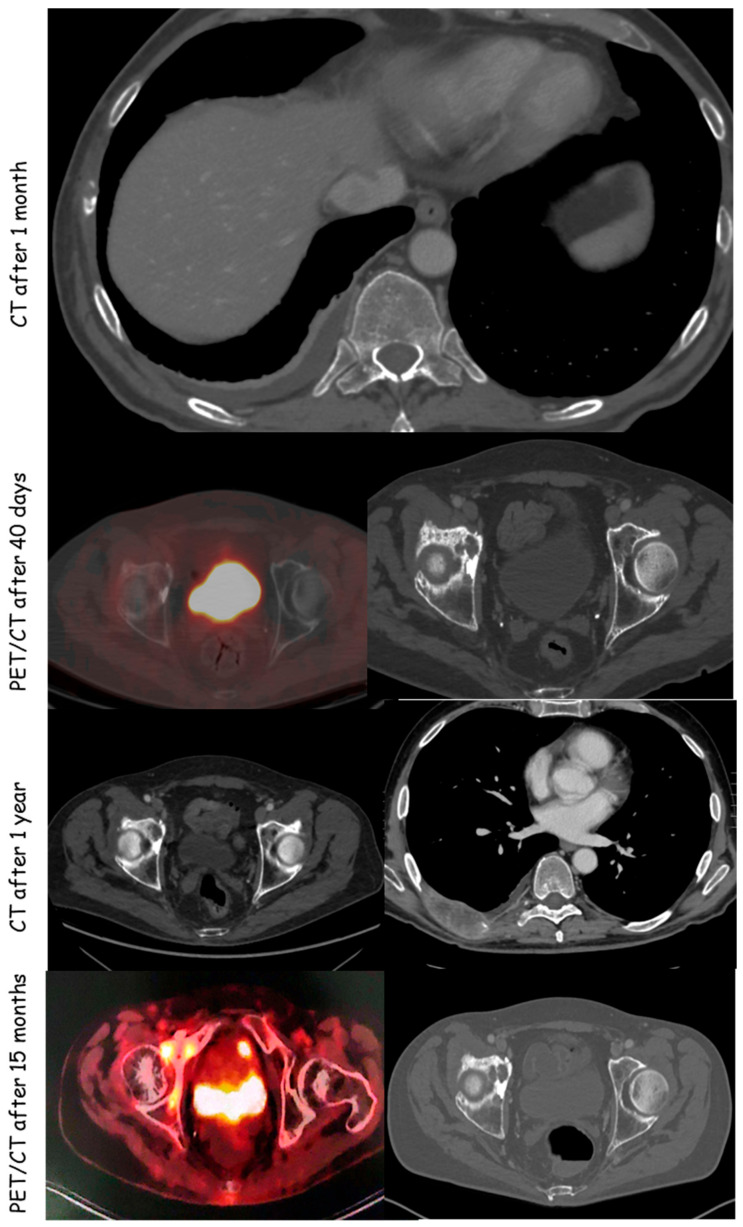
CT at 1 month and at 1 year from the treatment of cryotherapy with cementoplasty. PET/CT at 40 days and 15 months from above treatment.
